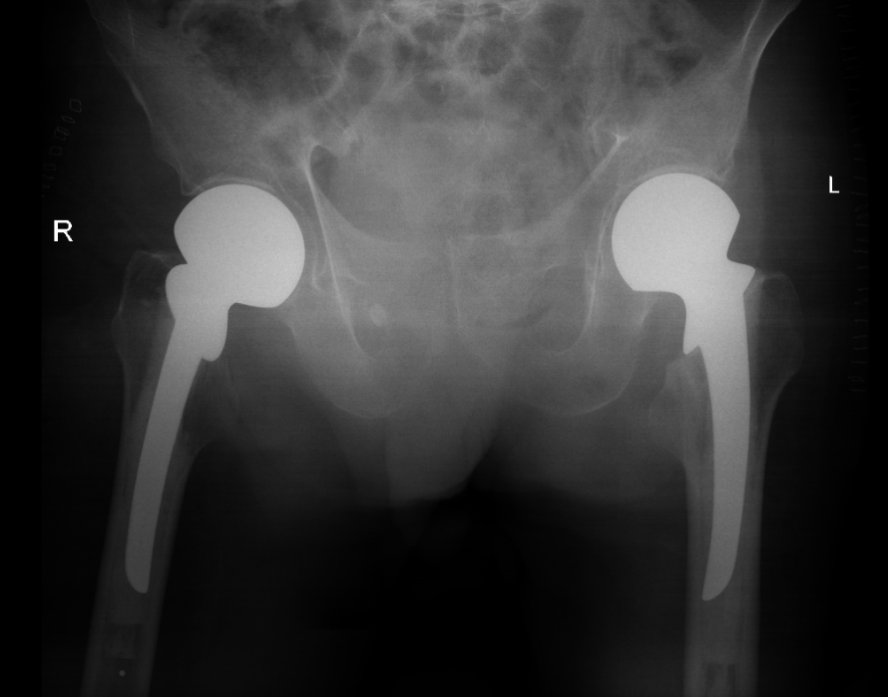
Anterioposterior radiograph of the pelvis showing bilateral fractures of the neck of femur treated by cemented Thompson hemiarthroplasties.
